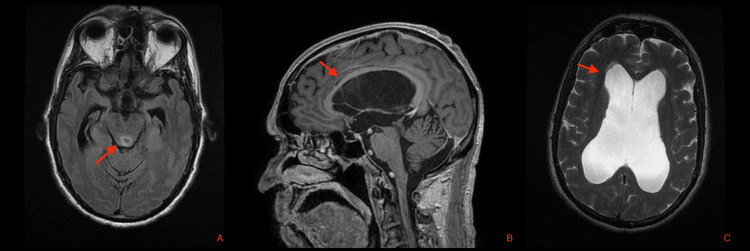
Preoperative brain MRI findings. (A) Axial FLAIR sequence showing an expansive lesion in the tectal plate (red arrow) compressing the cerebral aqueduct. (B) Sagittal T1-weighted contrast-enhanced image showing supratentorial ventricular enlargement consistent with obstructive hydrocephalus (red arrow). (C) Axial T2-weighted image demonstrating supratentorial hydrocephalus (red arrow). MRI: magnetic resonance imaging; FLAIR: fluid-attenuated inversion recovery
